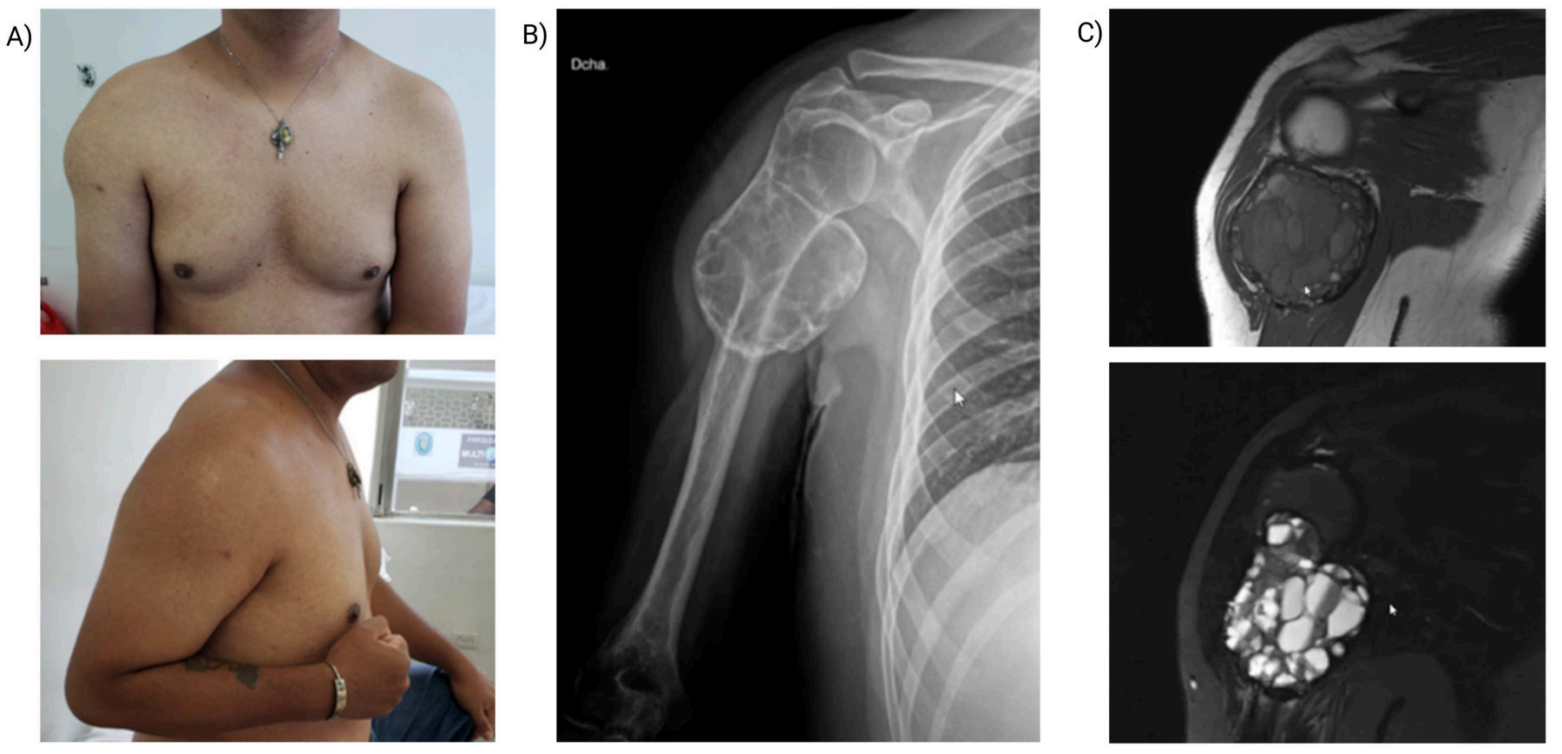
Preoperative patient evaluation. (A) Clinical image showing soft tissue displacement at the right proximal humerus. (B) Anteroposterior radiograph reveals a bony lesion in the posterolateral metaphysis of the proximal humerus with a bulging periosteal reaction, internal septations, and a soap-bubble appearance, characteristic of aneurysmal bone cyst (ABC). (C) Coronal MRI: T1-weighted image (top) shows soft tissue displacement and inflammatory changes in the deltoid muscle. T2-weighted image (bottom) demonstrates fluid levels within the lesion, consistent with ABC.
